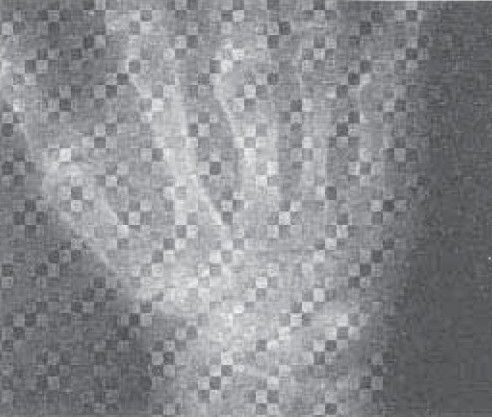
X-ray Left wrist and hand showing less osteolytic activity than previous film.
